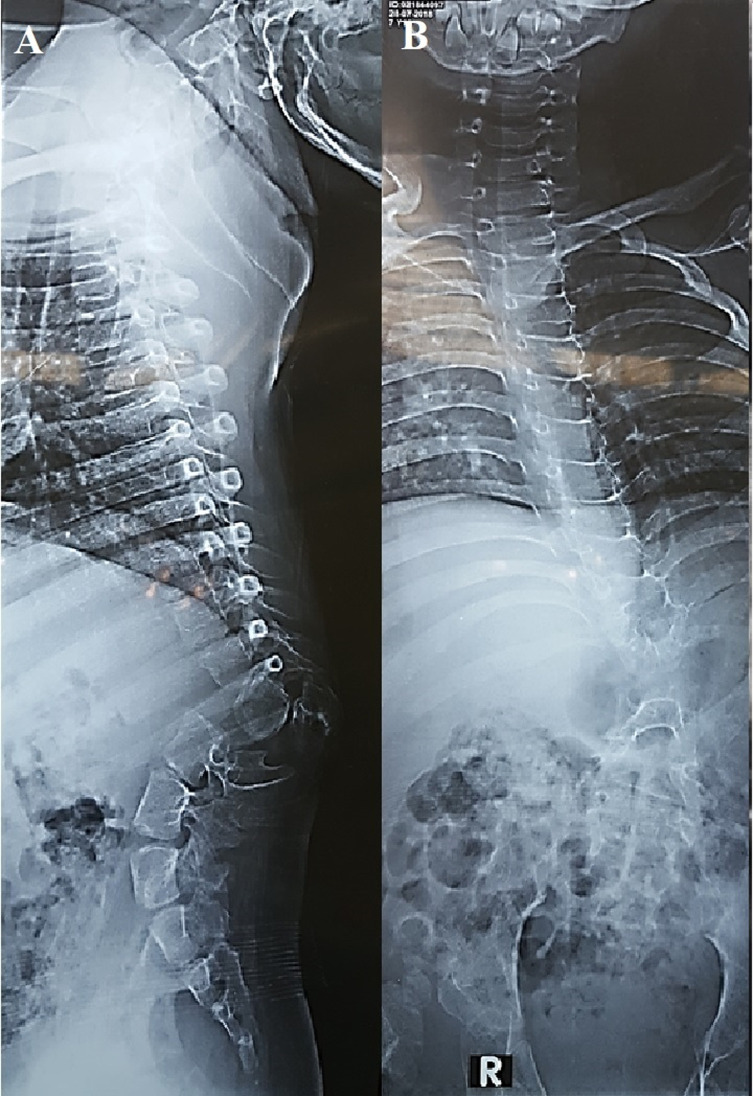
Preoperative. (A) Lateral plain X-ray showing acute kyphosis. (B) Anteroposterior plain X-ray showing coronal deformity.
